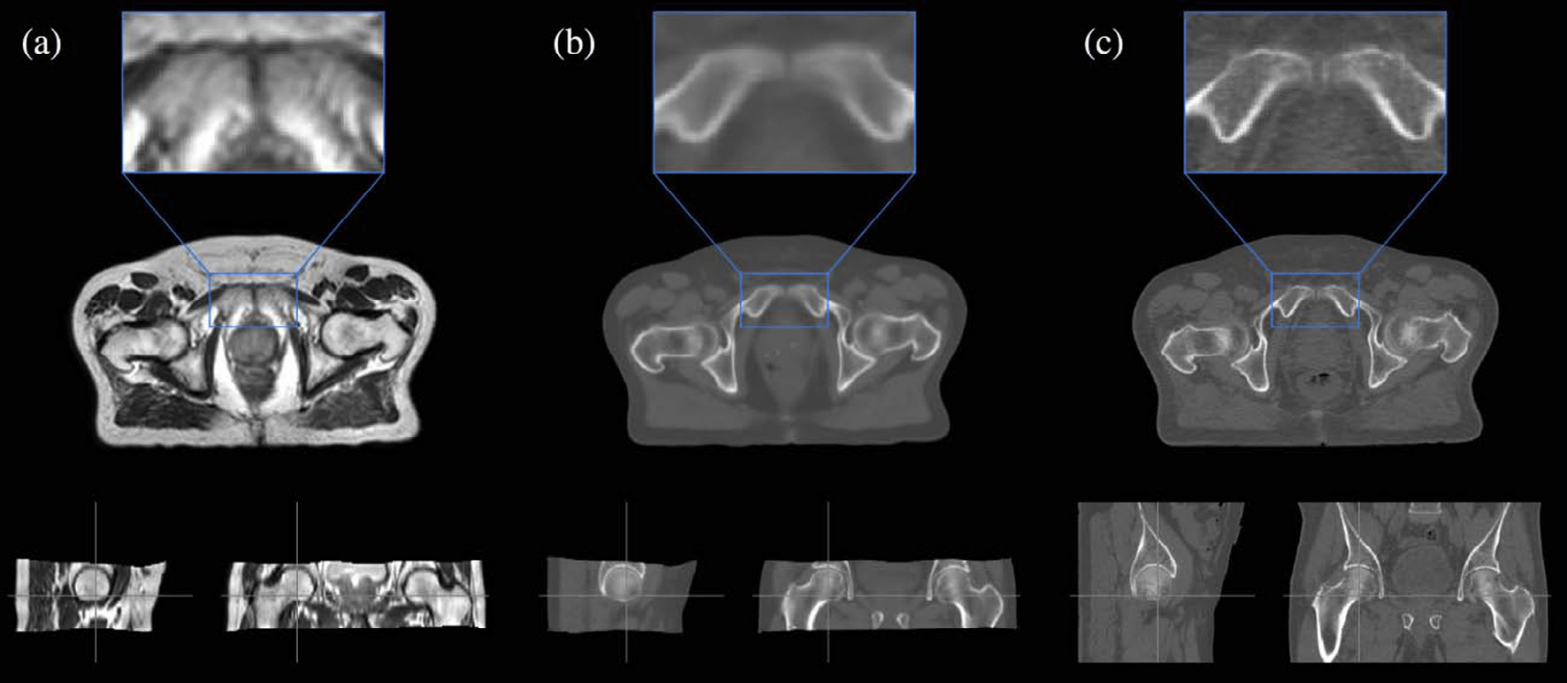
Sagittal, coronal and transverse plane images for a representative prostate patient showing (a) the MRI, (b) the sCT_a_ and (c) the planning CT. MR and sCT_a_ images were non-rigidly aligned to the planning CT for all patients.
